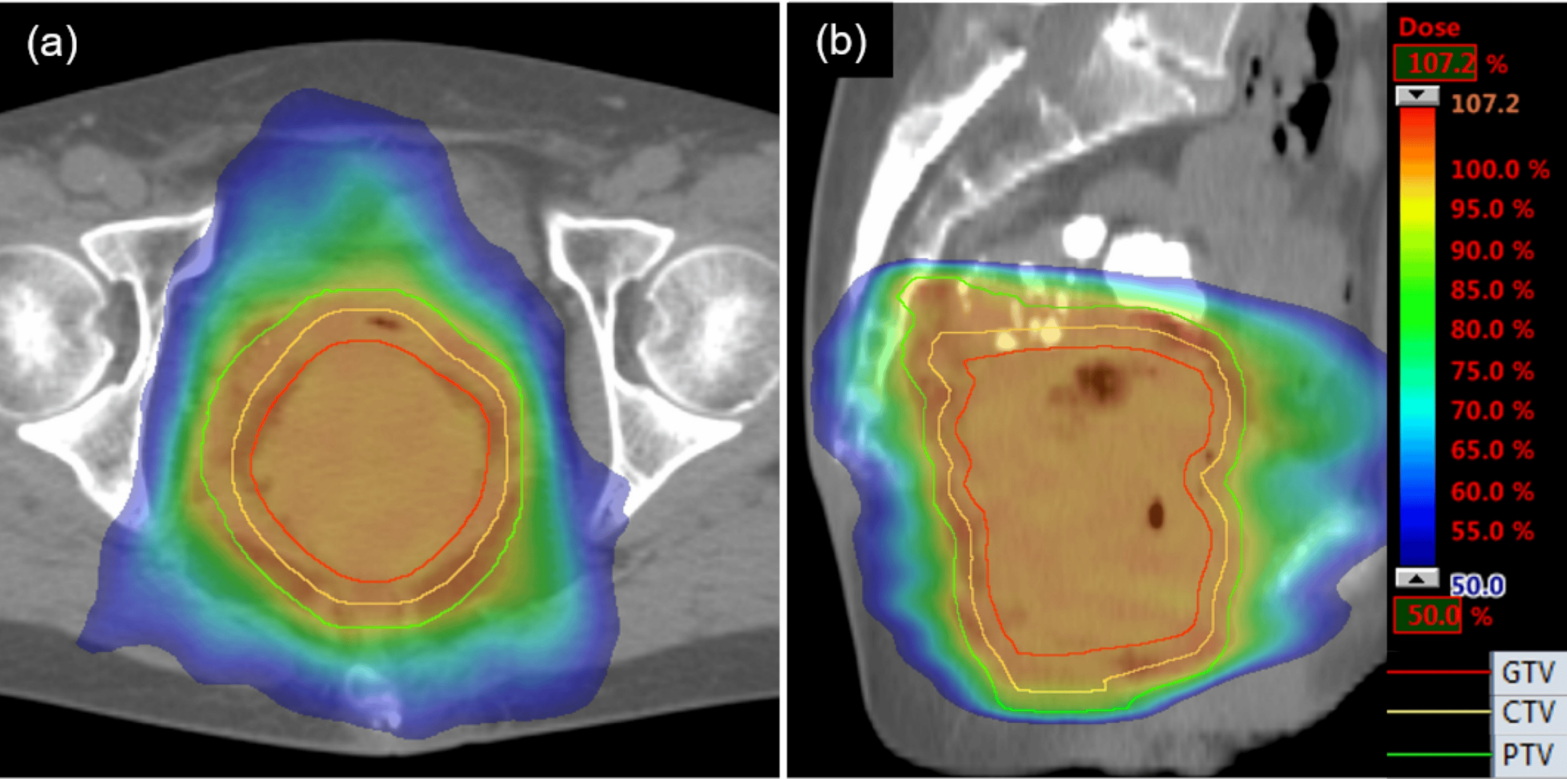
Images of dose distribution. (a) Axial view of CT. (b) Sagittal view of CT. CT: computed tomography; GTV: gross tumor volume; CTV: clinical target volume; PTV: planning target volume
